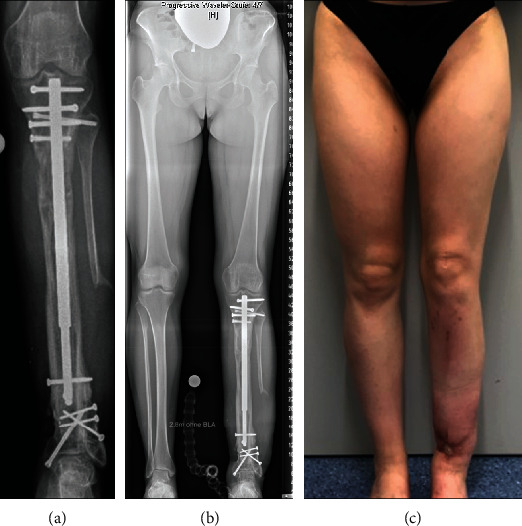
Patient 1. (a) Radiograph showing complete consolidation of the proximal tibial callotasis site after exchange to another lengthening nail for distraction. (b) Bilateral long-standing radiograph showing correct alignment and equalization of leg lengths. (c) Clinical presentation showing equal leg lengths.
